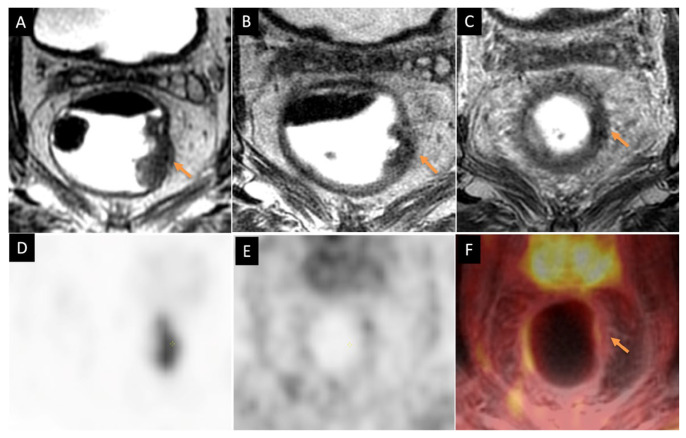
Rectal cancer treatment response assessment with PET/MRI. (A) Initial MRI showed a T3N1a rectal adenocarcinoma centered at the left mid rectal wall (arrow). (B) MRI after treatment with radiation therapy 25Gy in 5 fractions shows residual tumor with slight response (arrow). (C) MRI from PET/MRI exam after completion of total neoadjuvant therapy (8 cycles of FOLFOX) shows possible complete response and heterogeneous scar in the left wall (arrow). (D) Corresponding dedicated pelvic PET only image acquired for 15 min shows focal activity prior to treatment. (E) Post treatment dedicated PET only image acquired for 15 min shows no focal activity at the previous tumor site. (F) Fused PET/MRI (15 min PET bed fused to post contrast T1-weighted MRI) indicated complete response at the previous tumor site (arrow).
